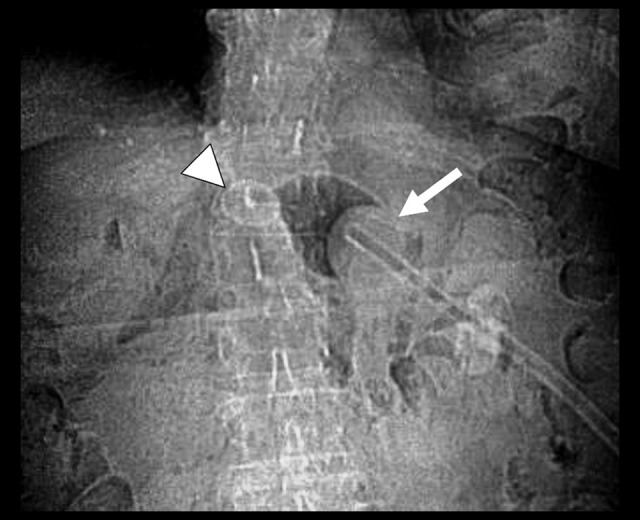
Abdominal radiograph demonstrates a round, radiodense disc in the mid abdomen, not connected to the balloon-retained PEG tube.
